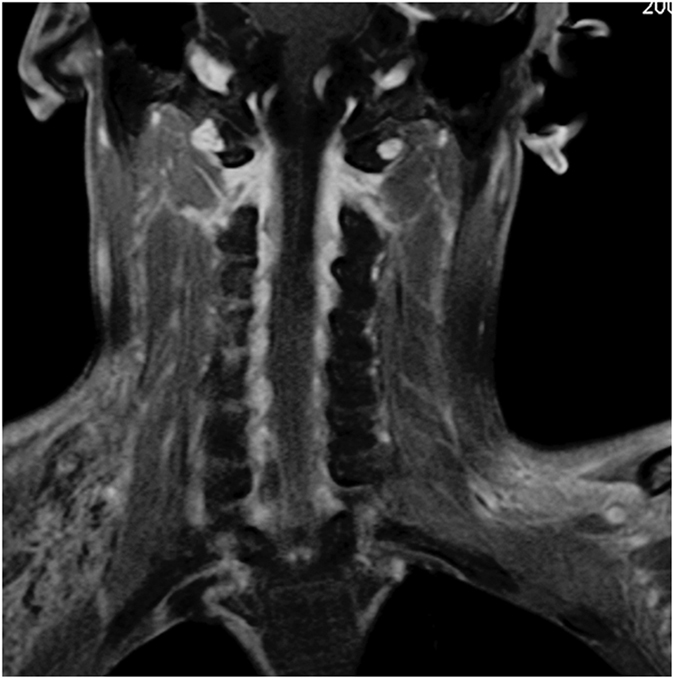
Coronal view of MRI visualizing the ventral rootlets. The number and size of rootlets and the connection with the cord are well visualized.
